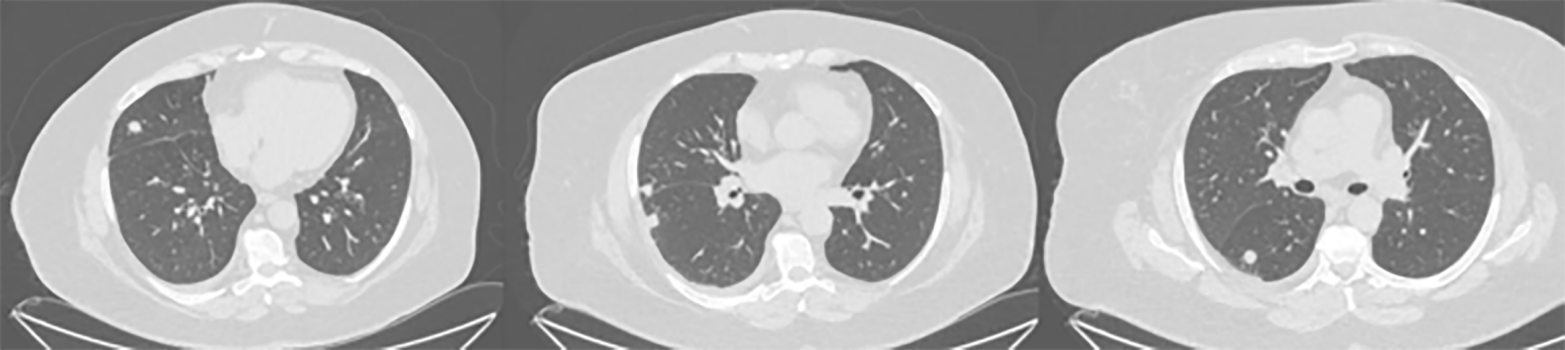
A 63-year-old woman with hypertension, obesity, and a history of smoking. A chest CT showed multiple nodules in the lungs. The patient was diagnosed with pulmonary histoplasmosis after the biopsy and positive serology.
